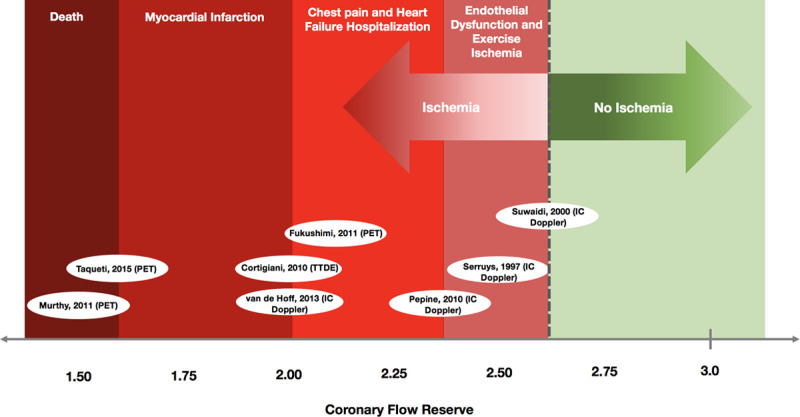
Coronary flow reserve (CFR) and a continuum of risk. The figure summarizes the relationship between CFR thresholds and the prognostic spectrum of cardiovascular outcomes, based on event rates from previously published studies. 3T CMR indicates 3-Tesla perfusion cardiac magnetic resonance imaging; IC Doppler, intracoronary Doppler studies; PET, position emission tomography; and TTDE, transthoracic dipyridamole echocardiography.^5–9,21–24^
